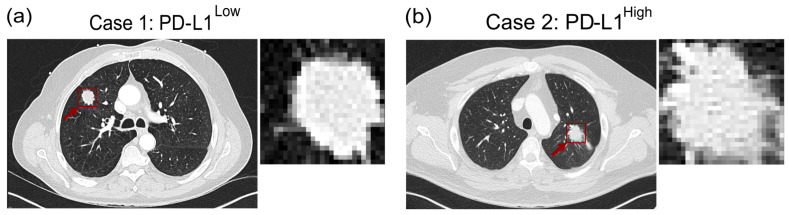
Representative cases with low and high PD-L1 expressions. (a) A pretreatment CT image of a PD-L1^High^ NSCLC (arrow) in the right lung. (b) A pretreatment CT image of a PD-L1^Low^ NSCLC (arrow) in the left lung.
